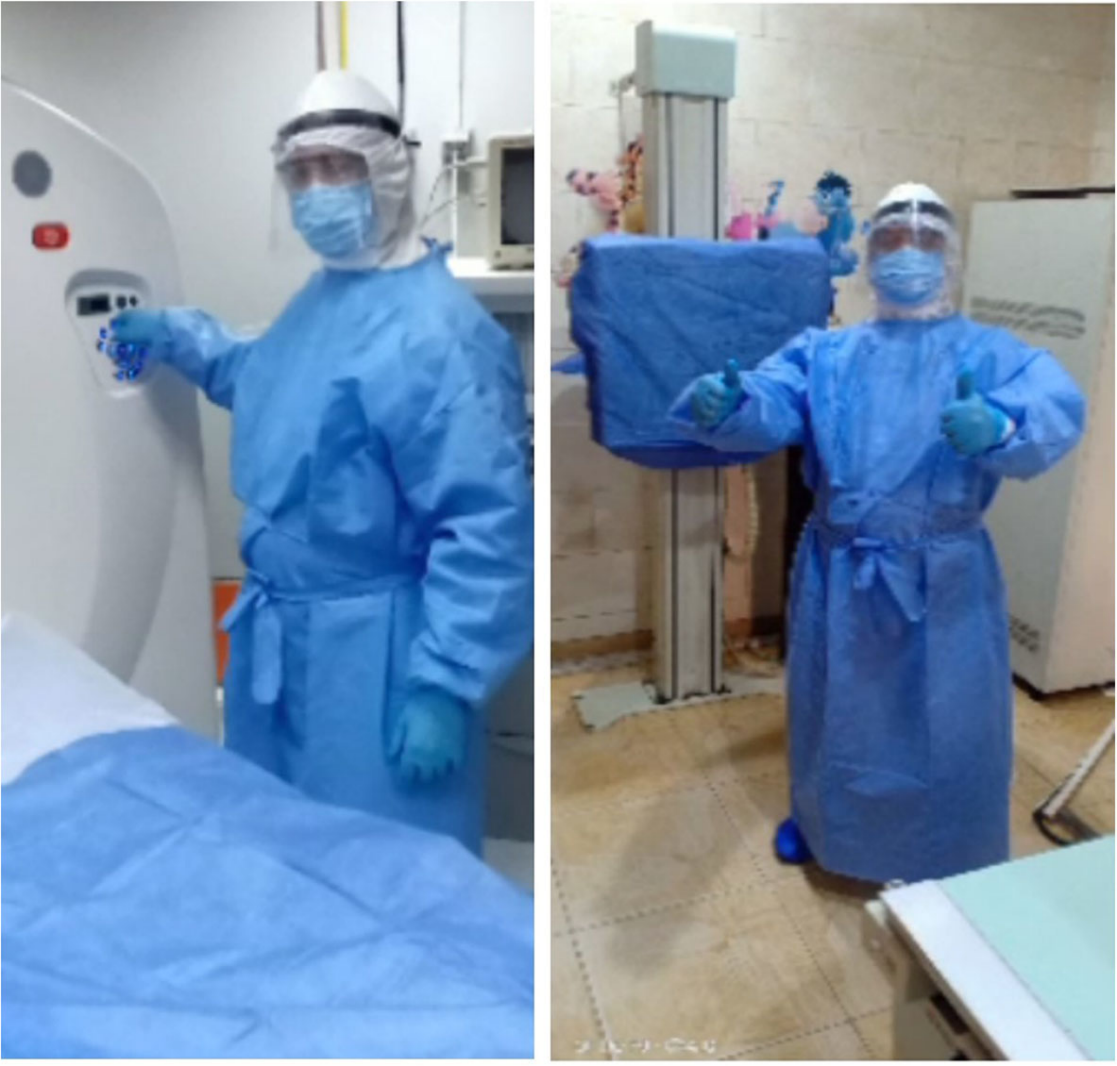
Protection in the imaging rooms: a technologist is wearing PPE and standing beside the CT gantry (a); and X-ray bucky (b). The Imaging table of the CT scanner and the X-ray bucky are covered with disposable surgical sheet
